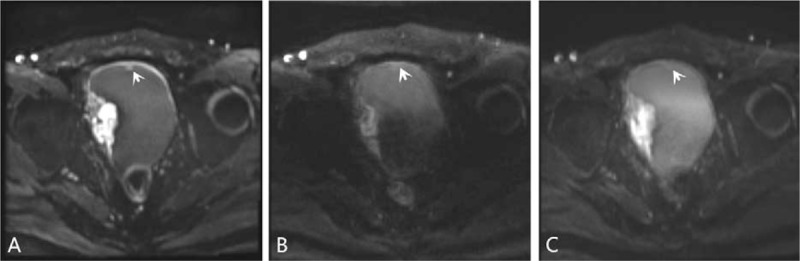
Comparison of (A) iShim, (B) RESOLVE, and (C) ss-EPI DWI (*b* = 1000 s/mm^2^) in a 66-year-old male patient with multiple bladder cancers. The smaller lesion located in anterior wall (size = 0.5 cm) (arrow) is clearly seen on (A) iShim. The same lesion could not be seen on (B) RESOLVE and the depiction of lesion is poor on (C) ss-EPI. Confidence score for smaller lesion on (A) iShim, (B) RESOLVE, and (C) ss-EPI is 3, 1, and 2. Images acquired with (B) RESOLVE and (C) ss-EPI DWI depict larger bladder cancer less clearly than in (A) iShim because of flow artifacts. The edge of lesion and normal bladder wall can be seen more clearly on (A) iShim DWI. Confidence score for larger lesion on (A) iShim, (B) RESOLVE, and (C) ss-EPI is 3, 2 and 2. iShim = integrated slice-specific dynamic shimming, RESOLVE = readout segmentation of long variable echo trains, ss-EPI = single-shot echo-planar-imaging.
